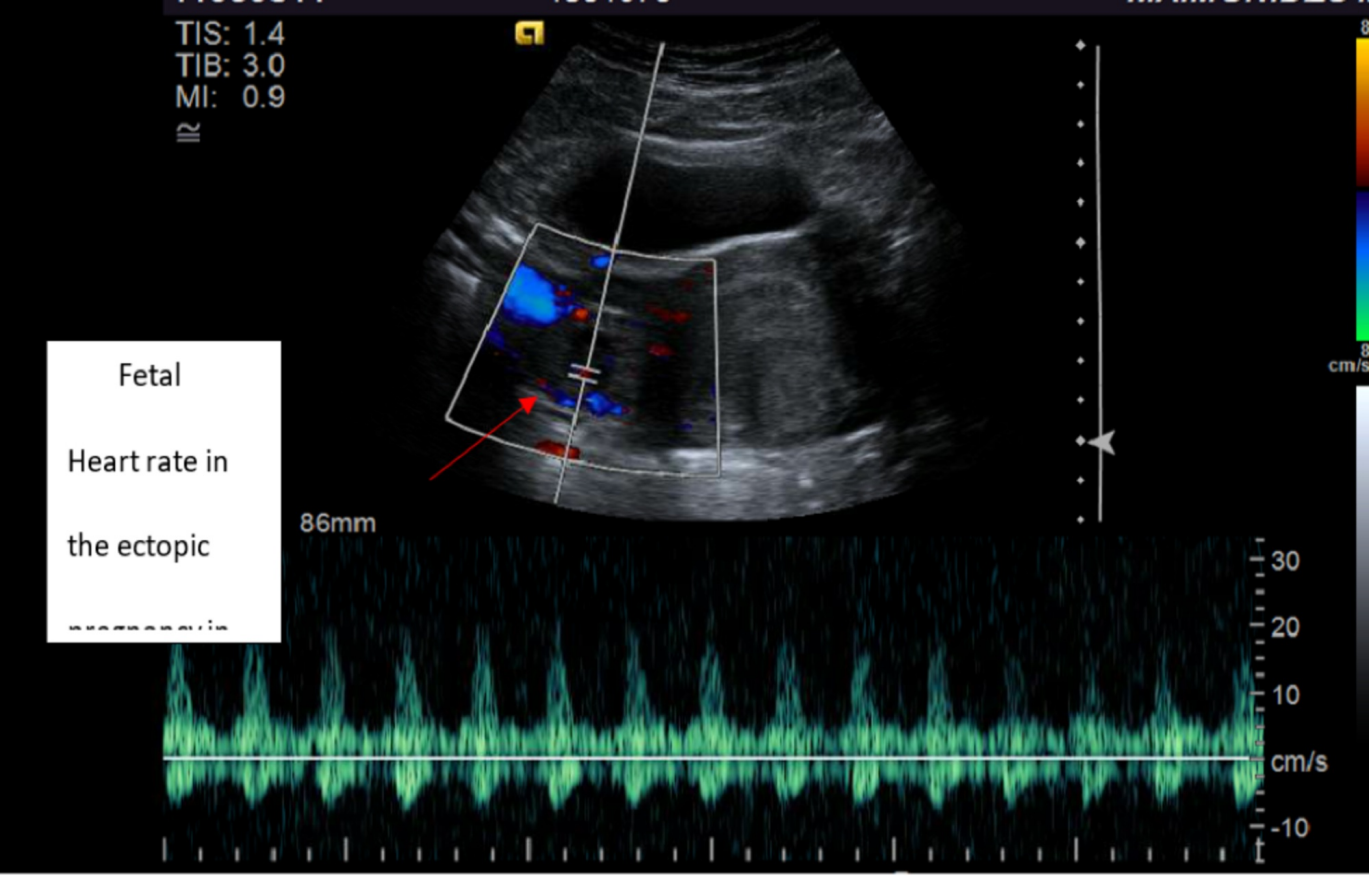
Pelvic ultrasound (transverse view): fetal heart rate demonstrated in the ectopic pregnancy in the right adnexal area
